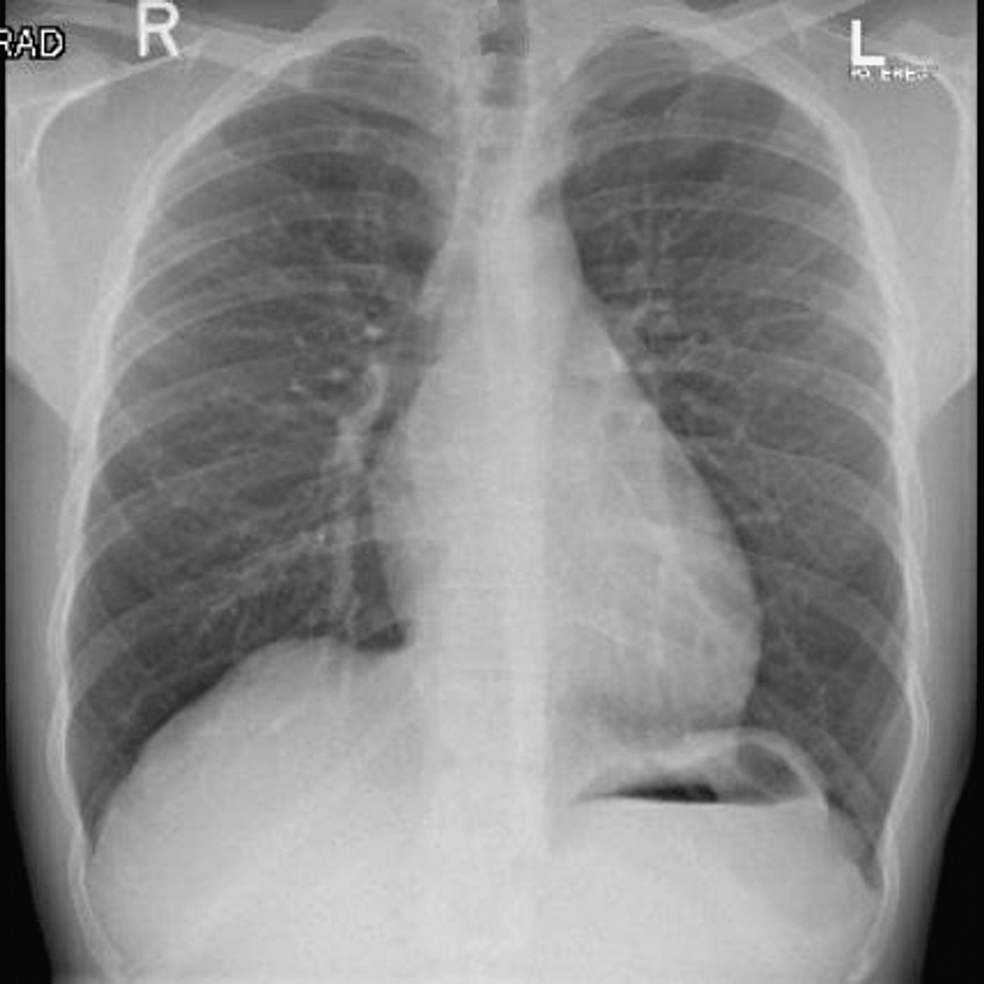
Chest X-ray with normal variation
